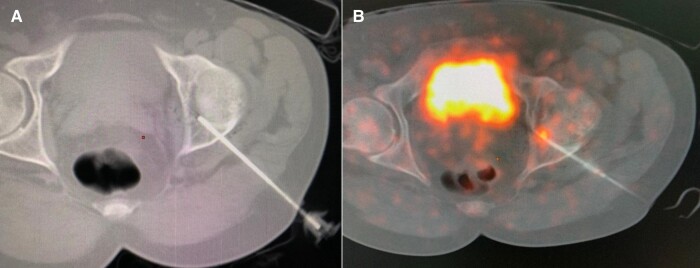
A, Computed tomography-guided radiofrequency ablation (RFA) needle in situ. B, Ga-68 DOTANOC PET/CT–guided RFA introducer needle tip placed at lesion tracer uptake site.
